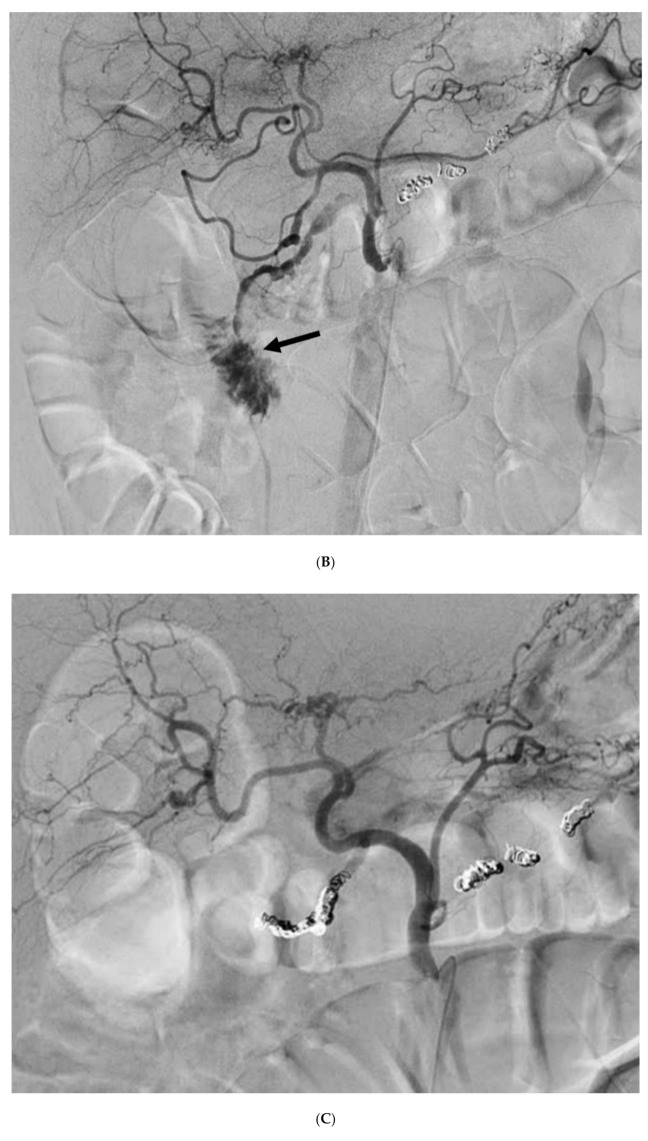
Transcatheter arterial embolization with coils. Angiographic images of a 55-year-old man with chronic pancreatitis. The patient had melena and endoscopic hemostasis was attempted, but the bleeding could not be stopped. (A) Contrast-enhanced CT was performed but a pseudoaneurysm was unclear by contrast-enhanced and three-dimensional CT. (B) The celiac axis angiogram revealed extravasation (indicated by the arrow) from the gastroduodenal artery. A microcatheter could be inserted into the artery over the pseudoaneurysm, and coils were placed between the distal and proximal sites of hemorrhage. (C) After embolization with a coil, hemostasis was obtained. The patient had no signs of recurrent bleeding or complications after transcatheter arterial embolization.
